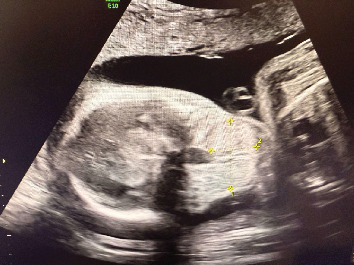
Imaging findings. 2D ultrasound of the fetus at 24 weeks gestation showing a fetal anterior neck mass.
